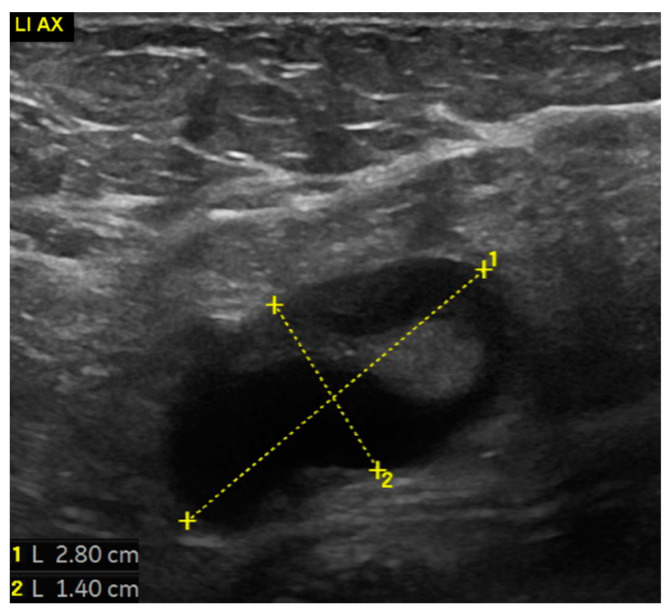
Ultrasonography (US) of the left axilla indicates lymphadenopathy. The lymph node measures 2.8 cm at its longest axis and 1.4 cm at its shortest axis, consistent with enlargement.
